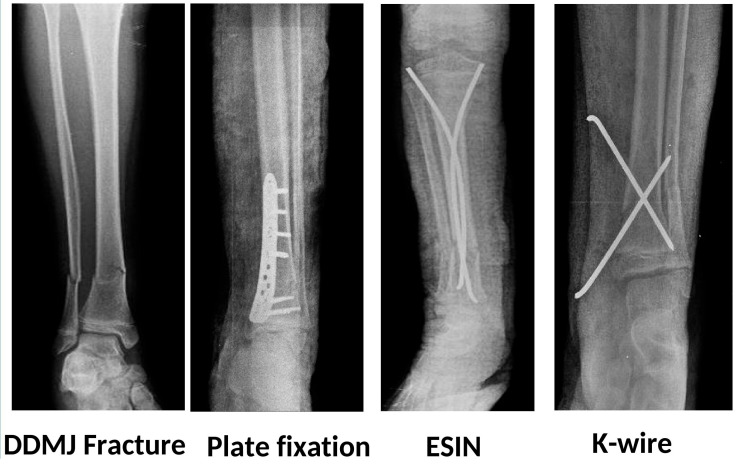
Radiographic images of patients who undergone plate fixation, ESIN, and K-wire fixation, respectively.
